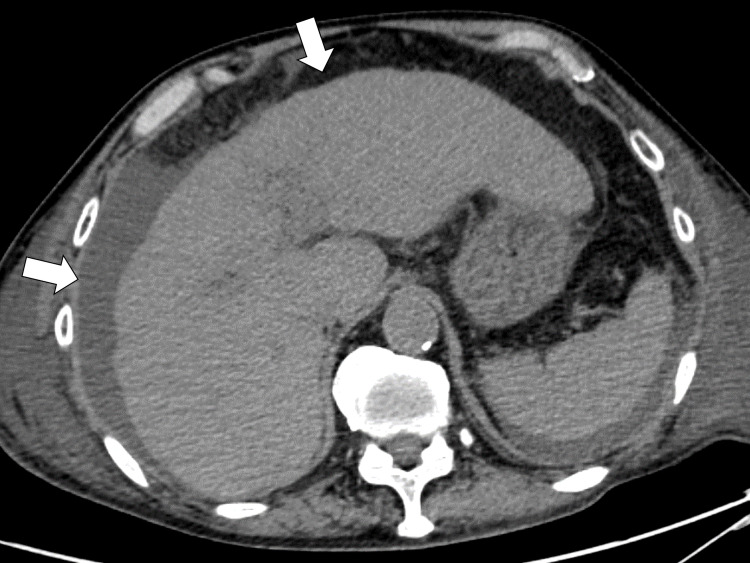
Abdominal CT showing liver cirrhosis with ascites accumulation on the surface of the liver (arrows) CT: computed tomography
